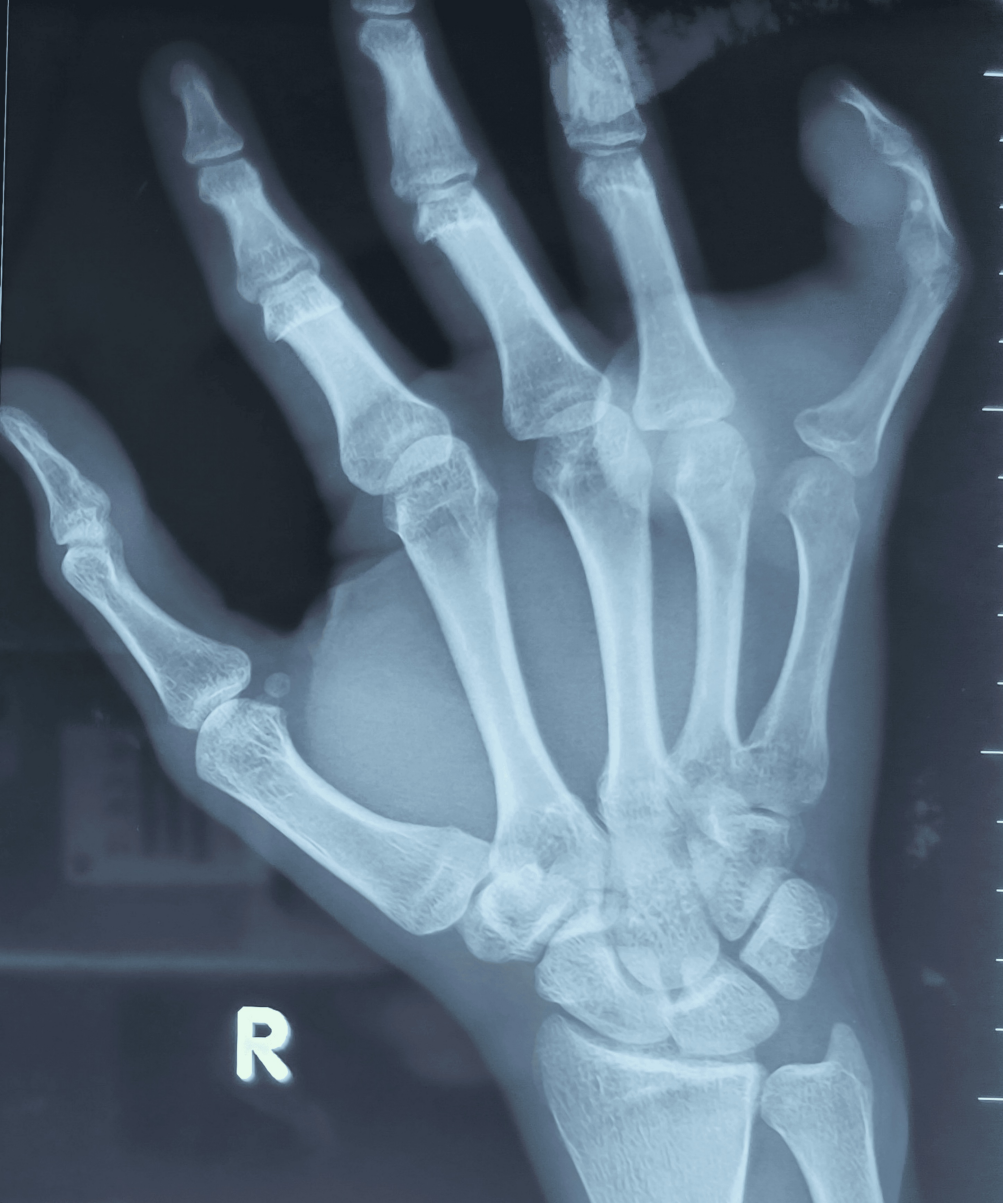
Plain X-ray of the right hand X-ray of the right hand showed significant soft tissue swelling but no sign of bony indentation or periosteal reaction
